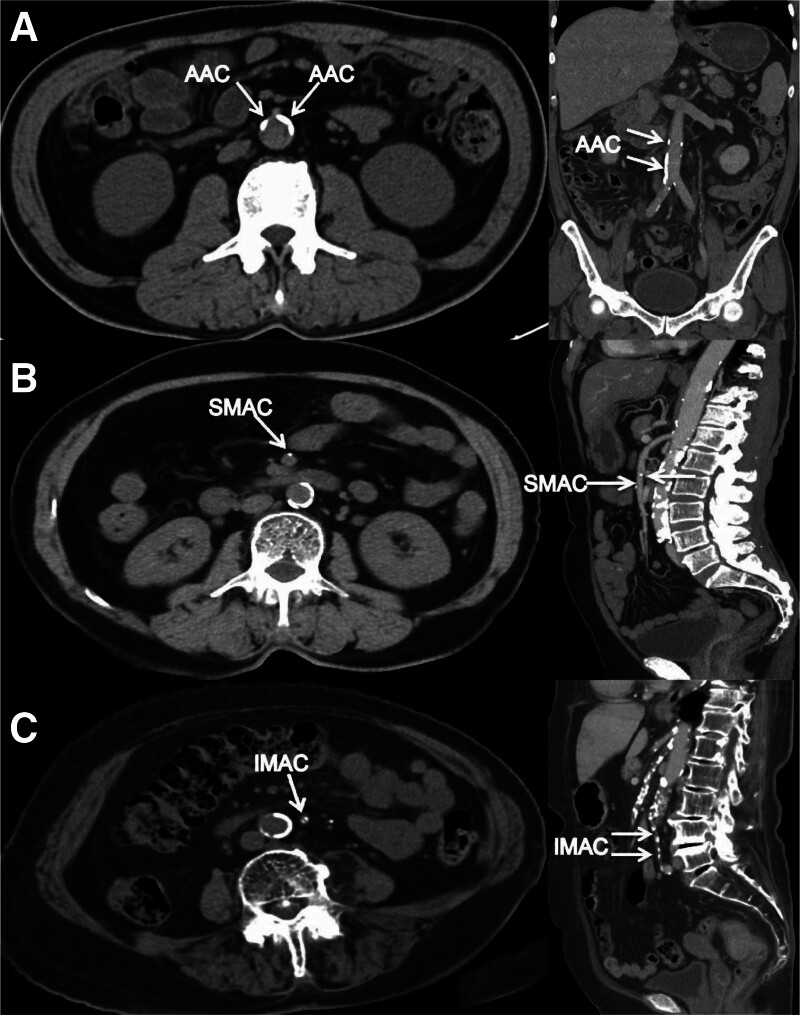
Examples of calcification on preoperative CT images in RC patients. (A) Image showing the AAC; (B) image showing the SMAC; (C) image showing the IMAC. AAC = abdominal aortic calcification; CT = computed tomography; IMAC = inferior mesenteric aortic calcification; RC = rectal cancer; SMAC = superior mesenteric aortic calcification.
